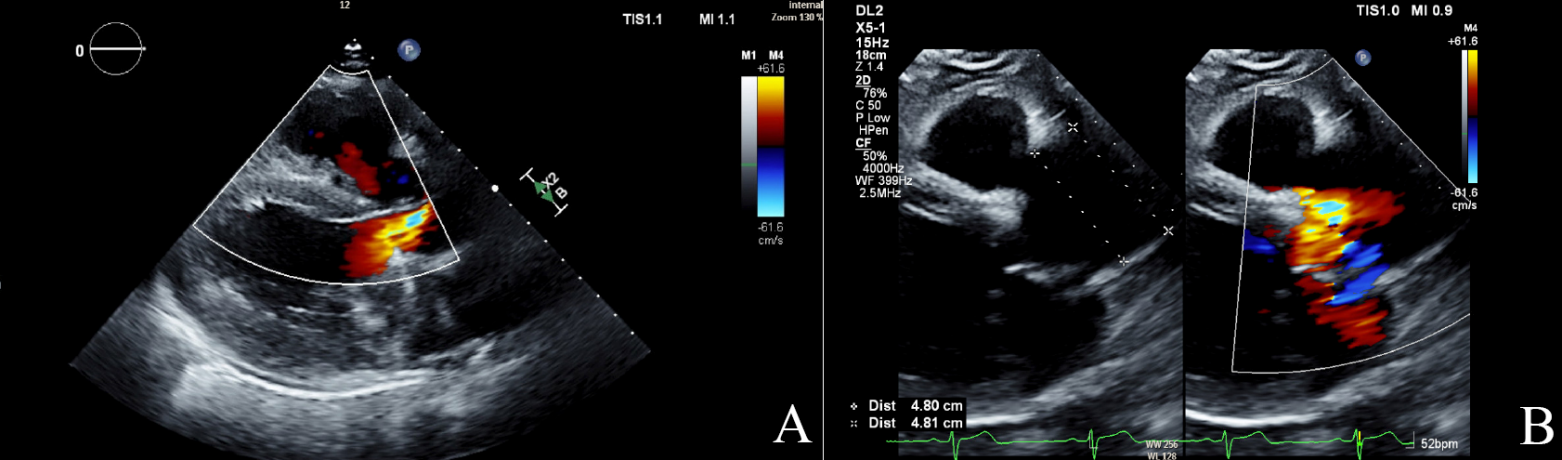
Echocardiographic findings in aortic regurgitation. (A) Color
